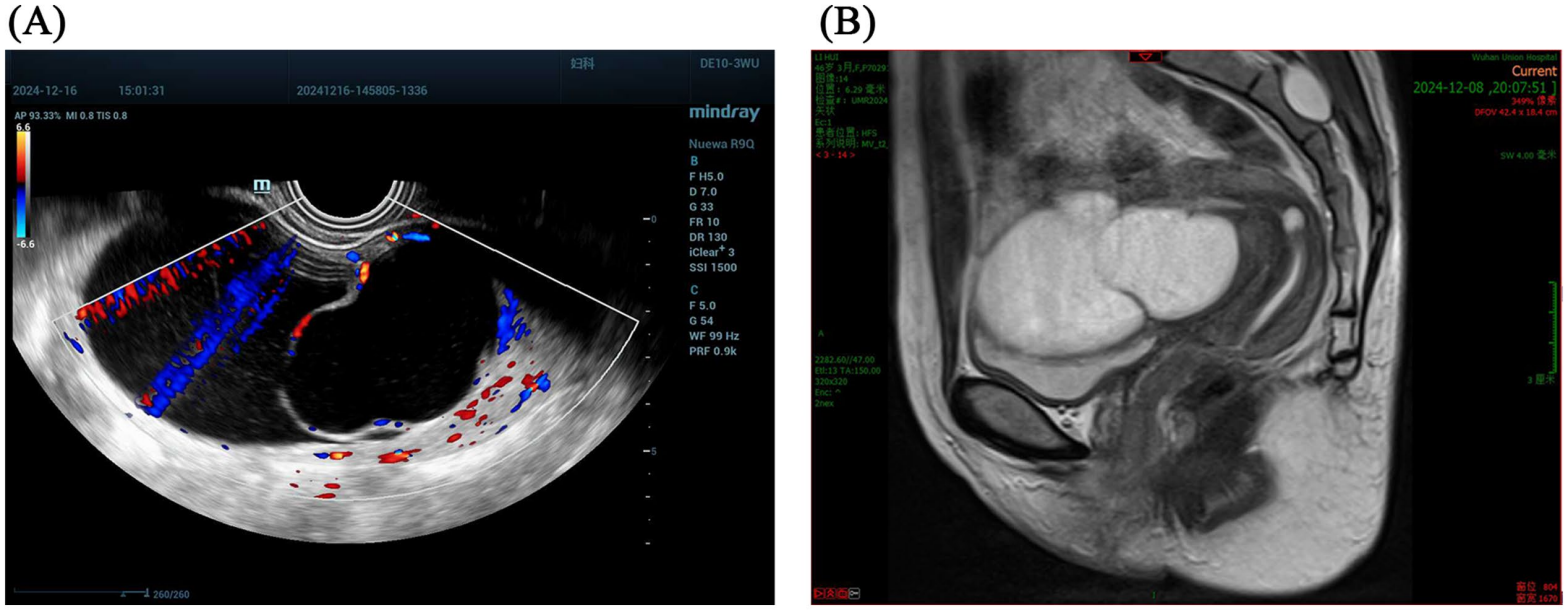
(A) Transvagina1 color Doppler ultrasound identified a hypoechoic mass characterized by well-defined margins and internal septations, with Doppler imaging showing blood flow. (B) Pelvic MRI confirmed the presence of a cystic structure in the anterior part of the uterine corpus.
